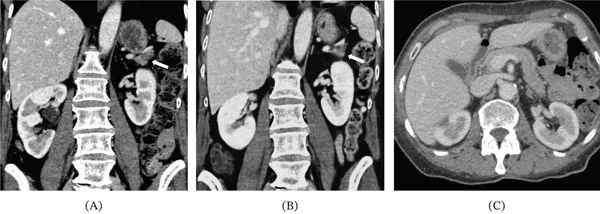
Follow‐up imaging at (A) 2 months and (B,C) 4 months after the completion of steroid treatment. CT abdomen shows progressive decrease in the size of the pancreatic tail lesion (arrows) and near‐complete resolution of renal lesions.
